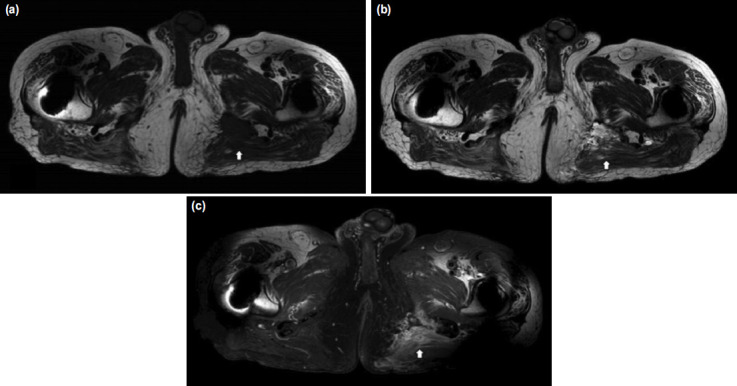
The axial image of the pelvis magnetic resonance imaging in a 79-year old male. (a) A cystic lesion (arrow) showing low signal intensity was observed in T1-weighted view. (b) It was observed that the same lesion (arrow) showed high signal intensity in T2-weighted view. (c) In the contrast-enhanced T1-weighted view, it is observed that the wall of the cystic lesion (arrow) is enhanced.
